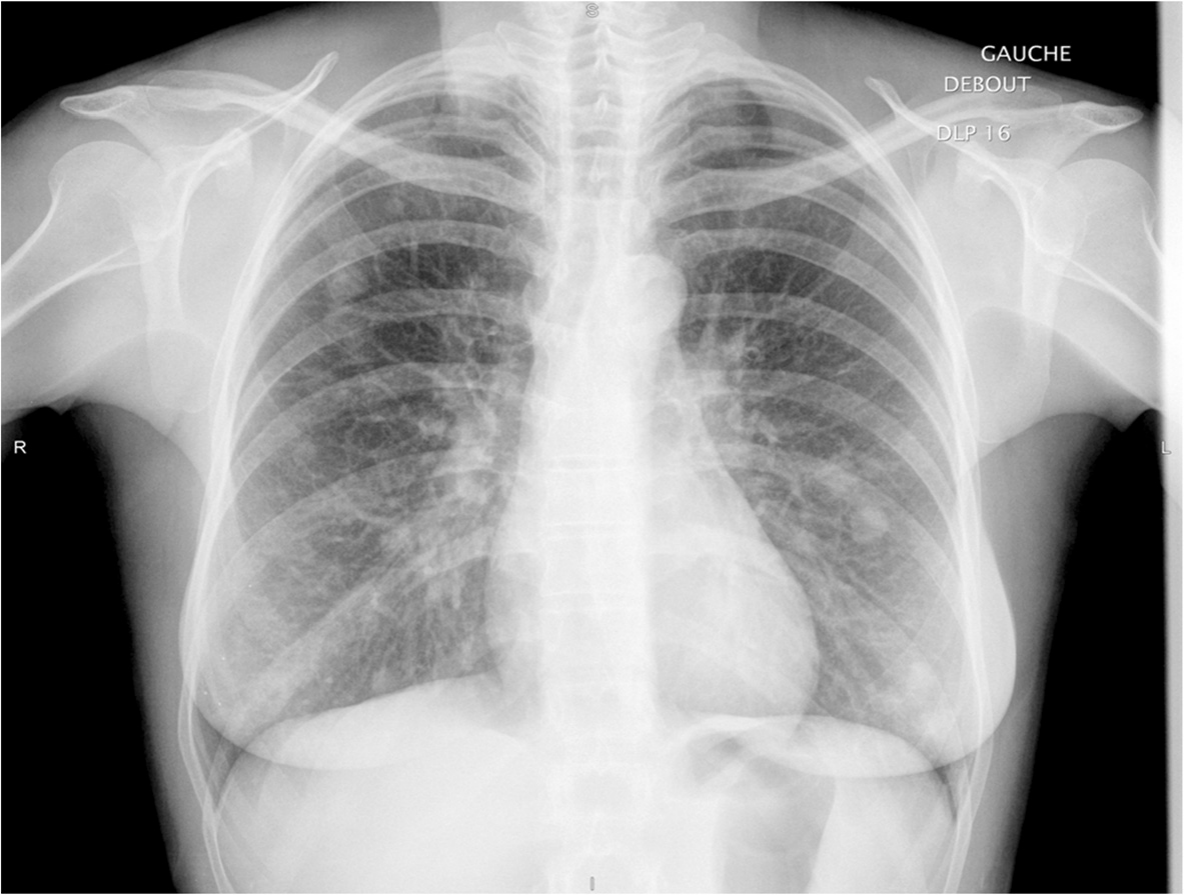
Chest radiography at the diagnosis.
